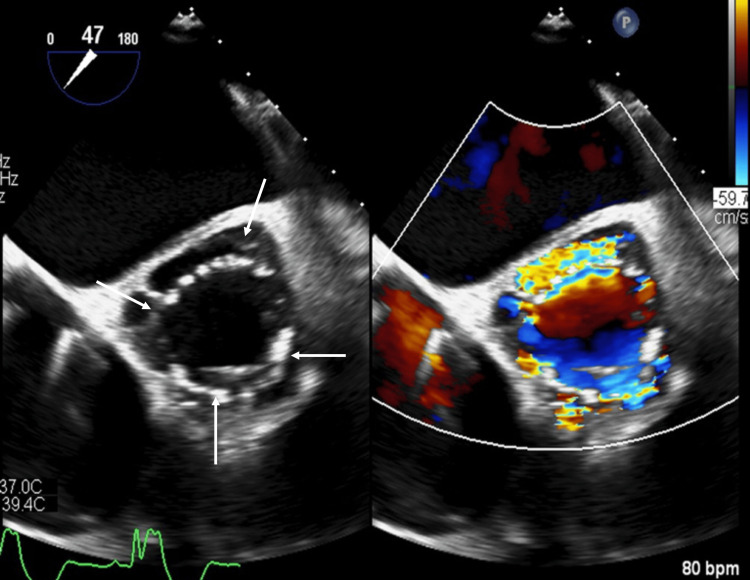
Transesophageal echocardiography shows contrast enhancement (arrows) around the aortic bioprosthesis similar to cardiac vascular enhancement on CTA, confirming the perivalvular abscess with aortic lumen communication turbulent flow inside on color Doppler images. CTA: CT angiography.
